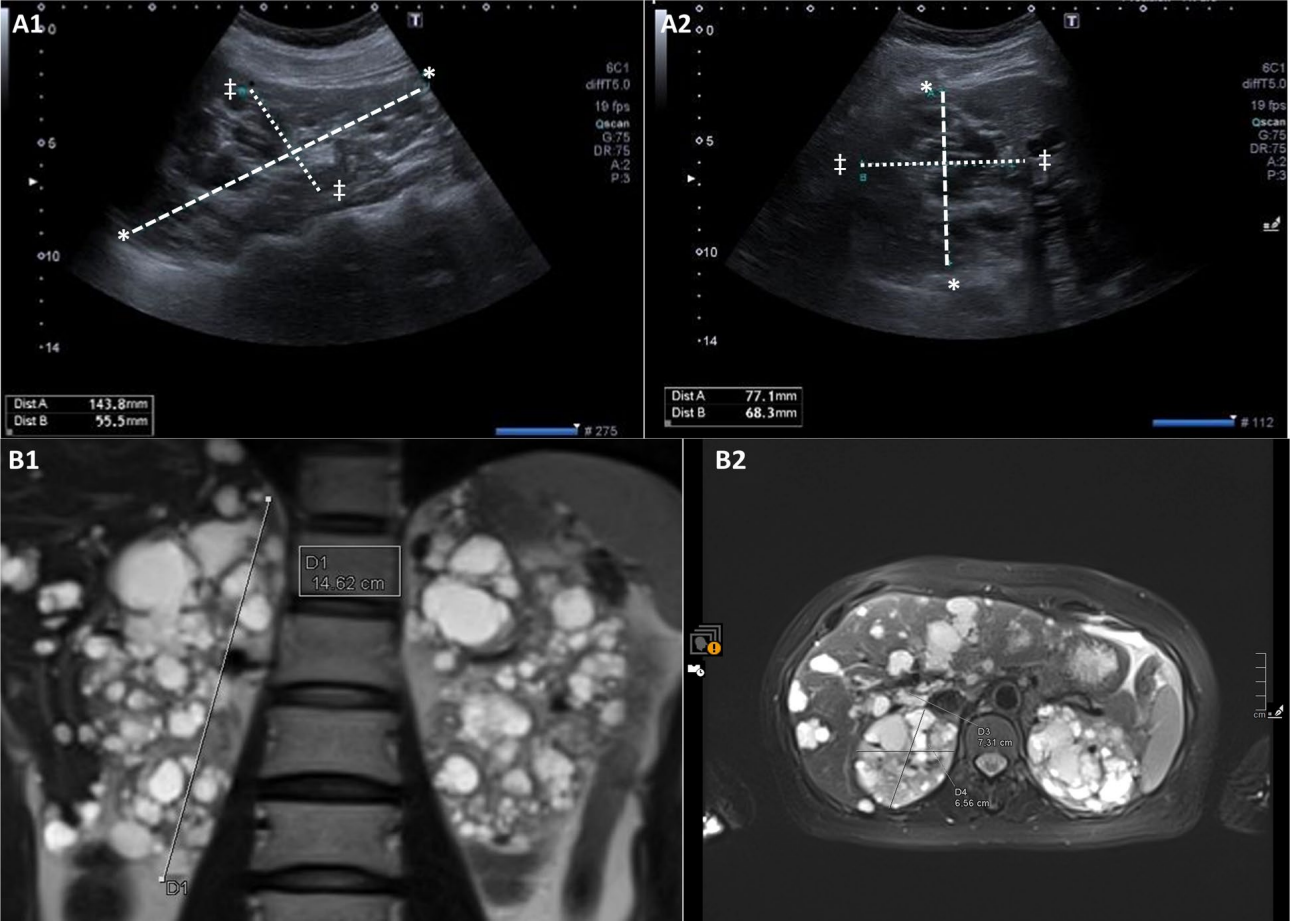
Kidney volume measurement of a 54-year-old female patient with autosomal-dominant polycystic kidney disease (ADPKD). A Kidney Volume Measurement Using Ultrasound. 1. Right Kidney Cranio-Caudal and Anterior–Posterior Diameters (Ellipsoid Formula). Cranio-Caudal Distance (marked * to *): 143.8 mm. Anterior–Posterior Distance (marked ‡ to ‡): 55.5 mm. 2. Right Kidney Antero-Posterior and Transverse Diameters (Ellipsoid Formula). Antero-Posterior Distance: 78.1 mm. Transverse Distance: 68.3 mm. B Kidney Volume Measurement Using Magnetic Resonance Imaging (MRI). 1. MRI T2 Coronal Cranio-Caudal Diameters (Ellipsoid Formula). Cranio-Caudal Distance D1: 146.2 mm. 2. MRI T2 Axial Anterior–Posterior and Transverse diameter for ellipsoid formula. Anterior–Posterior Distance D3: 73.1 mm. Transverse Distance D4: 65.6 mm
